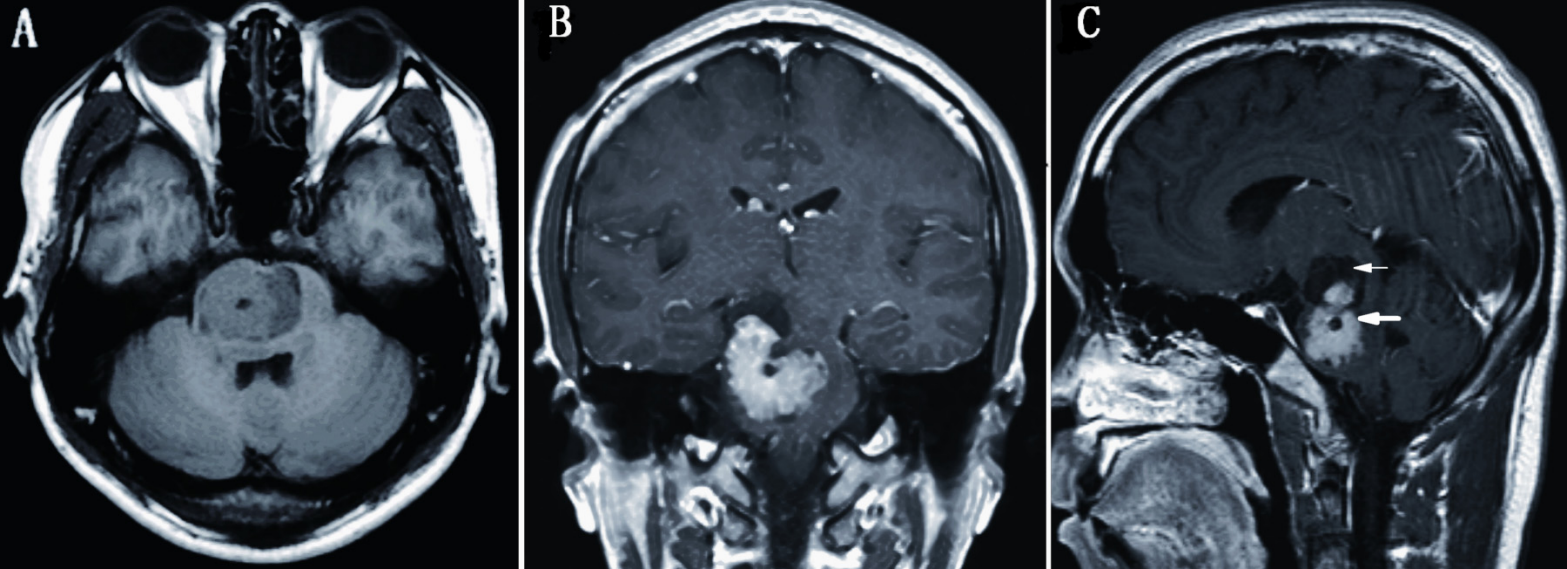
Preoperative magnetic resonance imaging demonstrating a cystic-solid mass of brainstem with mild edema. The solid part manifested homogeneous enhancement on T1-weighted gadolinium enhancement (thick arrow). The well-defined mass was mainly located in pons with the cystic part (thin arrow) extending to the right cerebral peduncle, but there was no evidence of attachment to dura mater. Axial T1-weighted imaging (A), coronal (B) and sagittal (C) post-contrast T1-weighted imaging.
